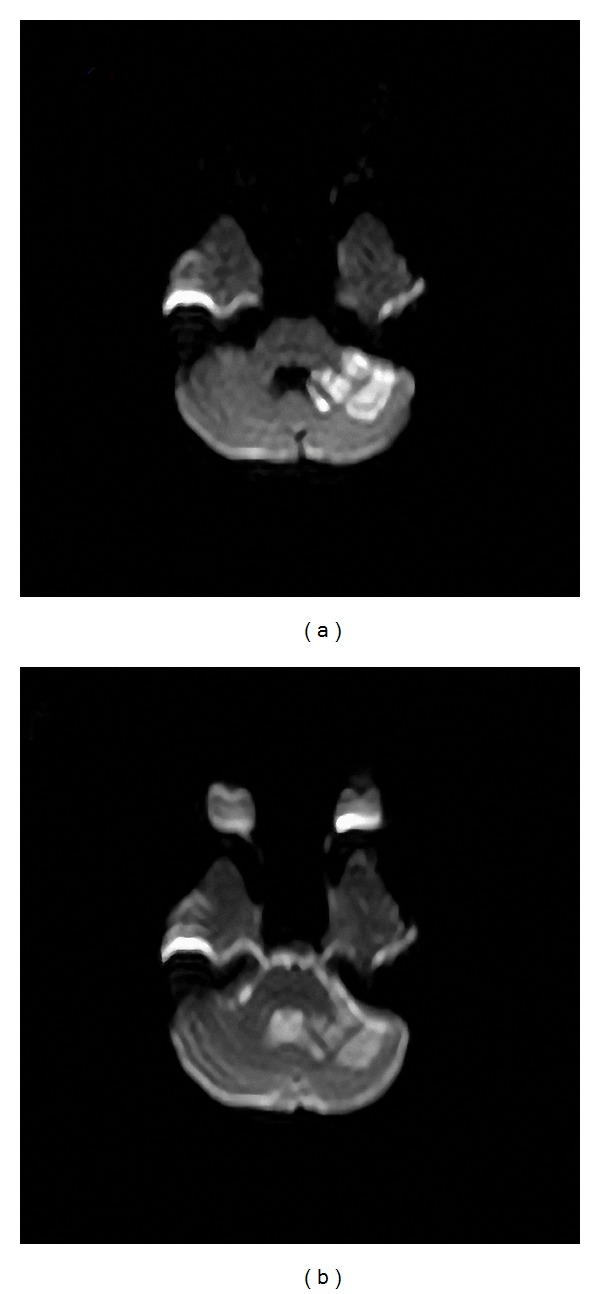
Brain diffusion MRI showing acute cerebellar infarction on the left cerebellar hemisphere.
